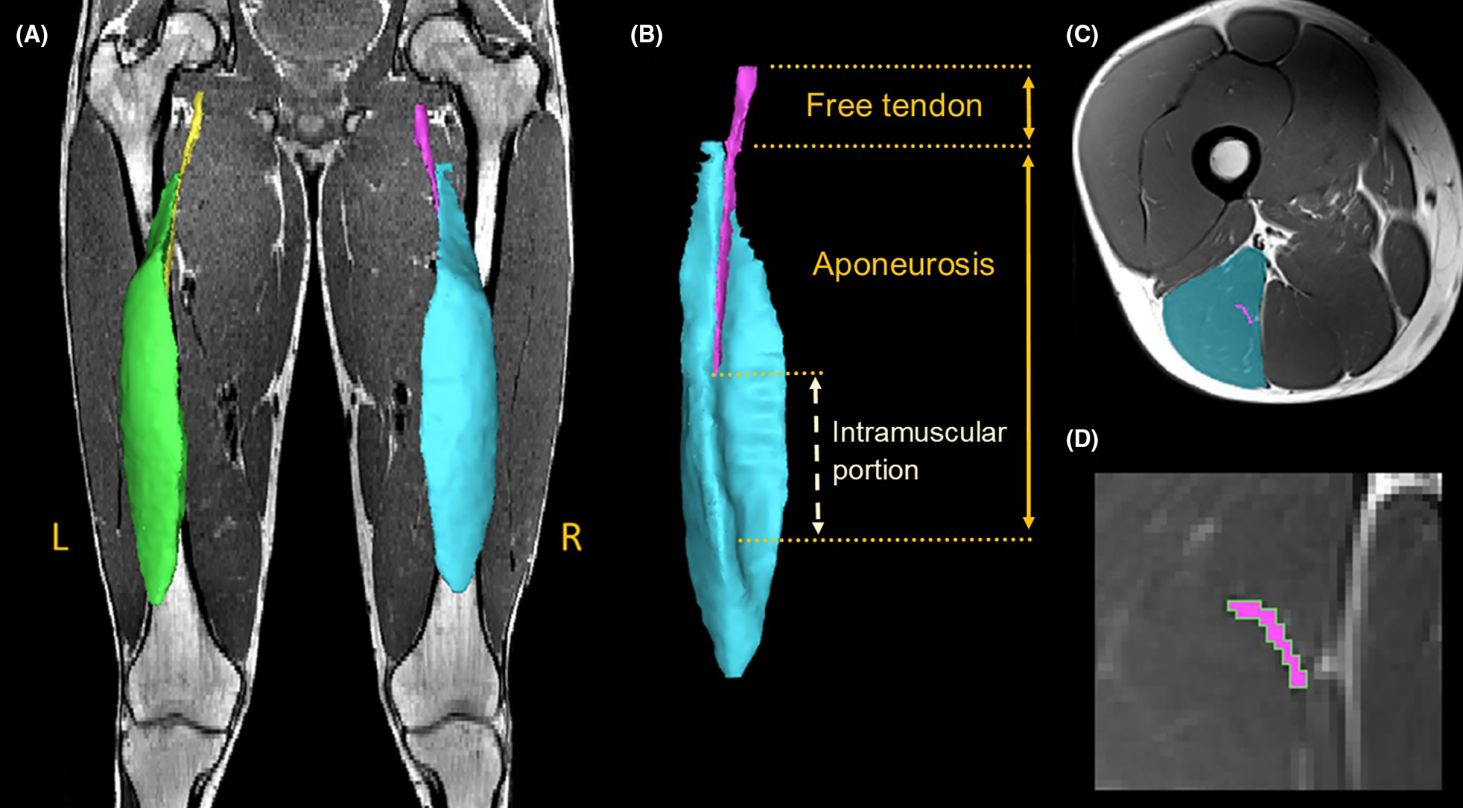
Muscle and tendon segmentation for a representative control participant. (A) Posterior view of a segmentation overlaying MRI, showing muscle tissue for the left (green) and right (blue) biceps femoris long head. Proximal tendon tissue is shown with the left (yellow) and right (purple) free tendon and a small proportion of aponeurosis visible. (B) Anteromedial view of the right BFlh whole MTU displaying muscle and proximal tendon tissue. (C) Axial plane image at approximately 50% of the muscle length of the right thigh with BFlh muscle and aponeurosis colored. (D) Magnified image showing the right BFlh aponeurosis (purple) with spline trace (green) of the interface area between muscle and aponeurosis.
